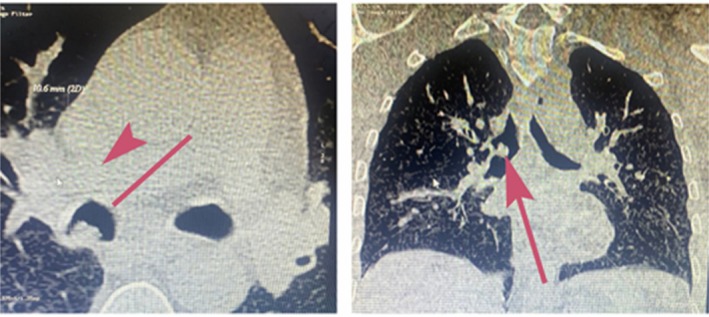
Computed tomography (CT scan) showing a lesion in the right bronchus (red arrow).
